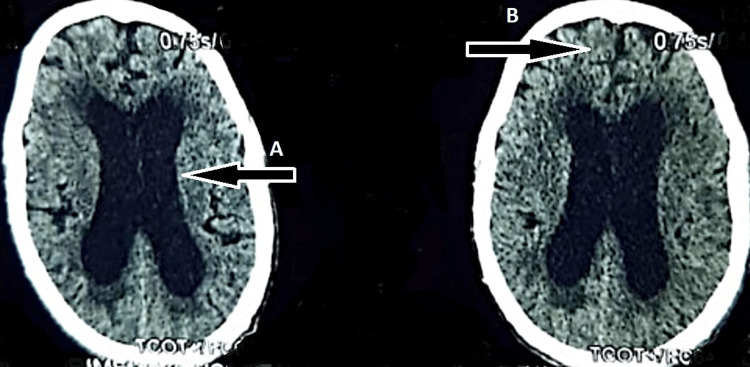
Axial CT brain demonstrating moderate dilatation of the lateral ventricular chain (Arrow A) and cortical atrophic changes mainly involving the bilateral frontotemporal regions in the form of prominent sulci (Arrow B)
